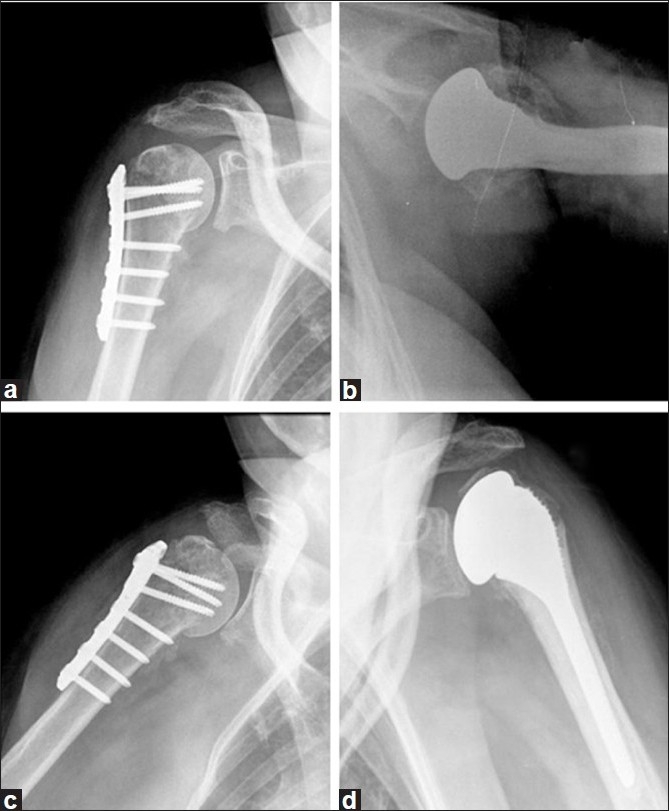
Radiographs of both shoulders 15 months after injury, showing consolidation of the right shoulder fracture (a,c) without signs of avascular necrosis, and good positioned hemiarthroplasty on the left shoulder (b,d) without evidence of loosening
